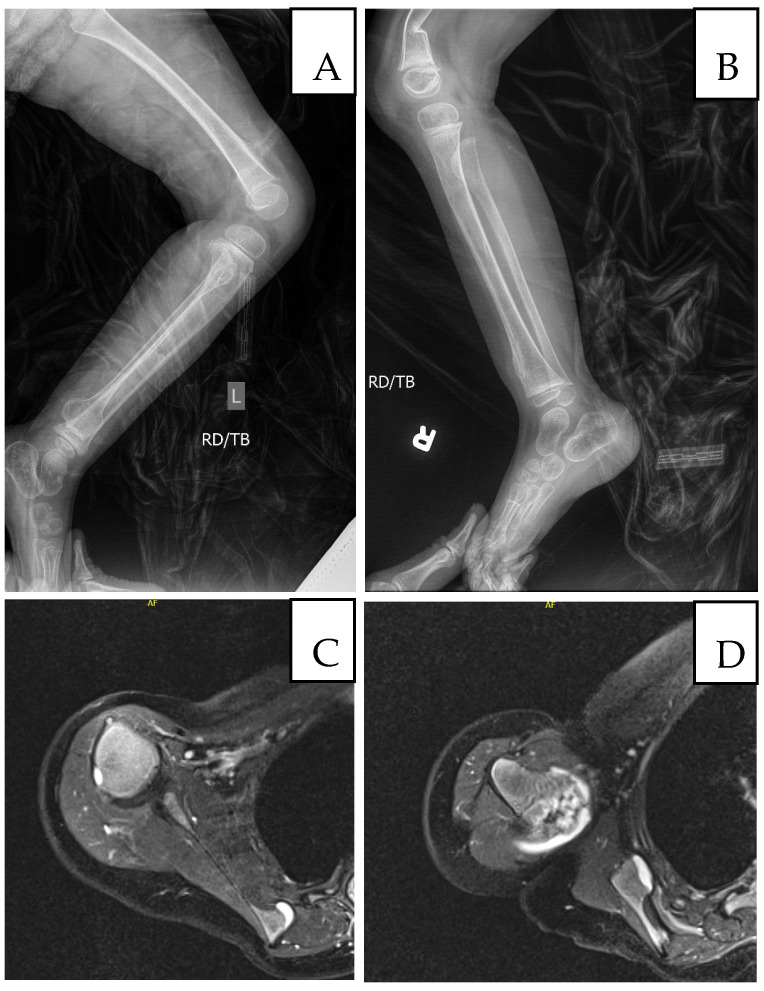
Imaging at age 5: (A) Left femur/tibia/fibula X-ray with sessile osteochondromas; (B) right tibia/fibula X-ray (comminuted distal right femur fracture shown); (C) MRI, medial border of left scapula; (D) MRI, head of left humerus and lateral left scapula.
